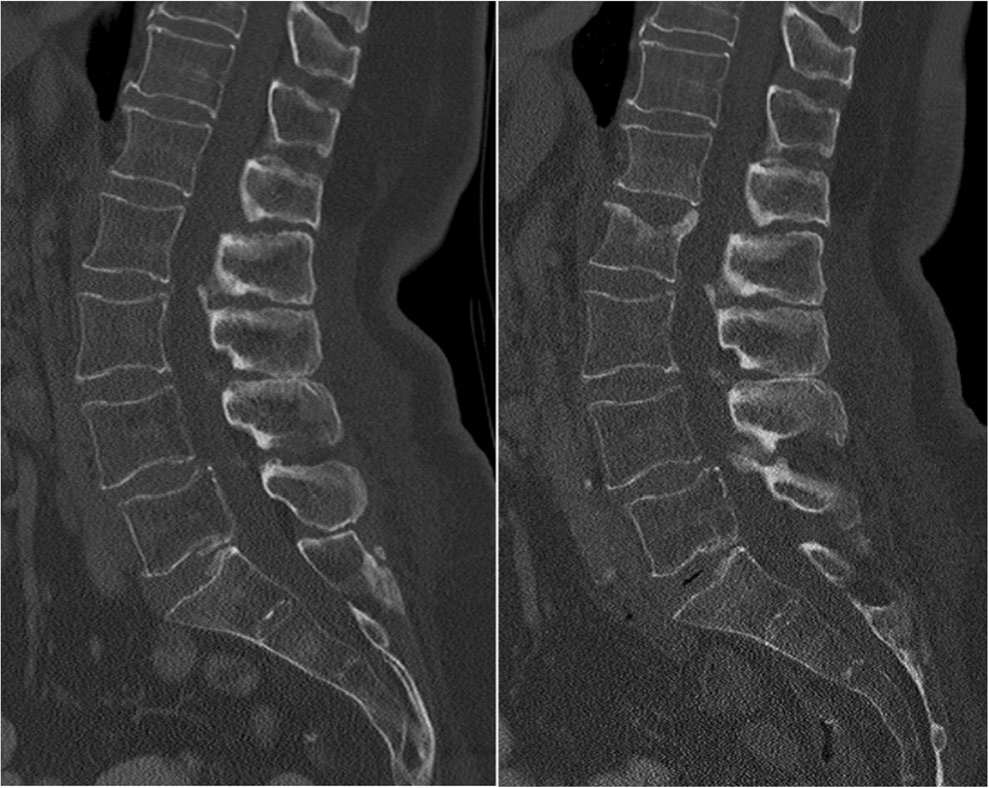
Left: baseline CT of a 72-year-old female patient with osteopenia according to DXA (*T* = − 1.7) and osteoporosis according to opportunistic QCT (BMD = 70.5 mg/cm^3^). Right: in follow-up after 5.2 years, the patient had sustained an incident vertebral compression fracture of L2
